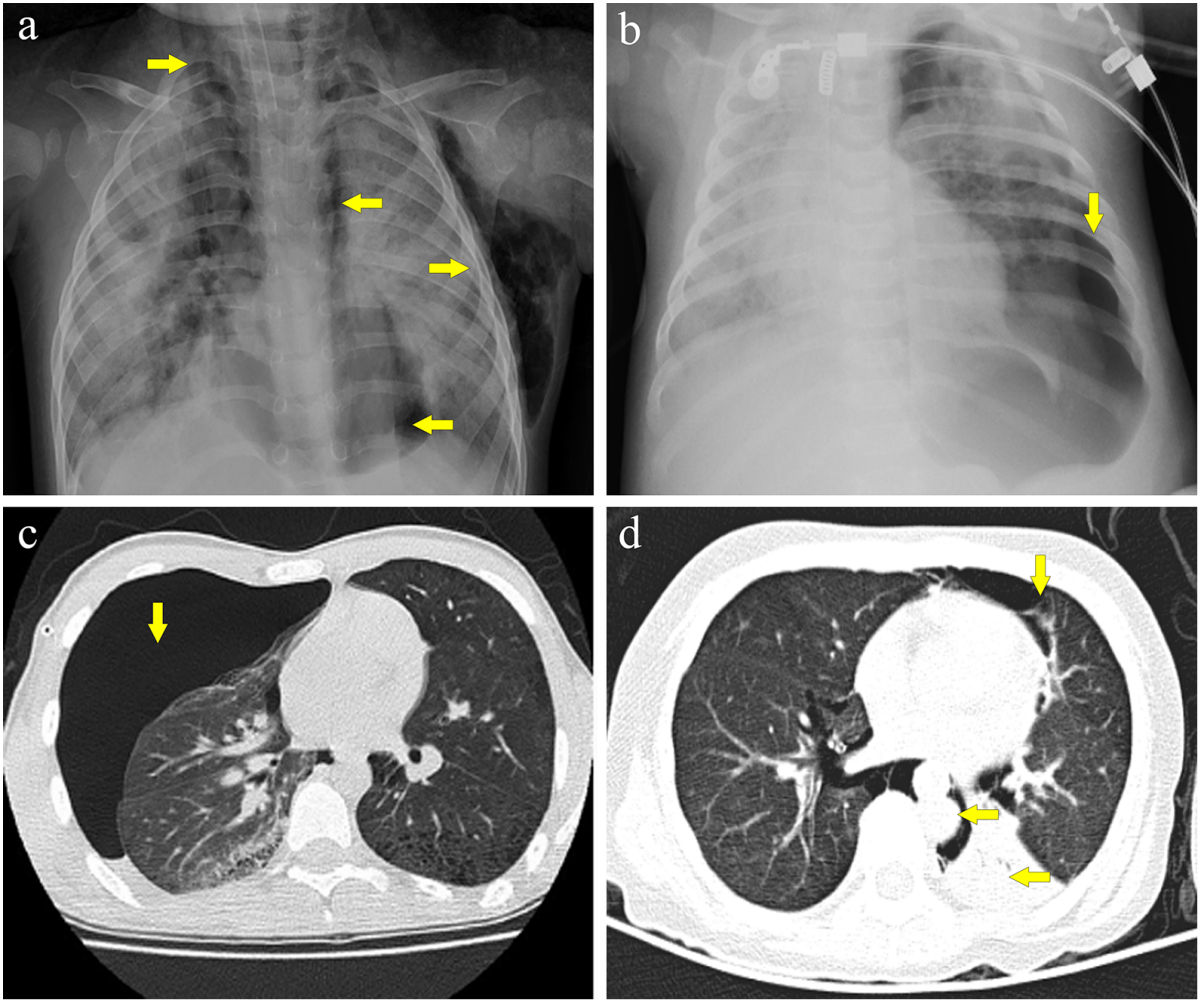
Imaging presentation. a Anterior–posterior chest radiograph of a 2-year-old patient showing subcutaneous emphysema and pneumomediastinum. b Anterior–posterior chest radiograph of a 3-month-old patient showing a left pneumothorax. c High-resolution computed tomography scan of a 3-year-old patient showing a right pneumothorax. d High-resolution computed tomography scan of a 10-year-old patient showing pneumomediastinum and pneumothorax. The right arrow indicates subcutaneous emphysema, the downward arrow indicates pneumothorax, and the left arrow indicates pneumomediastinum
